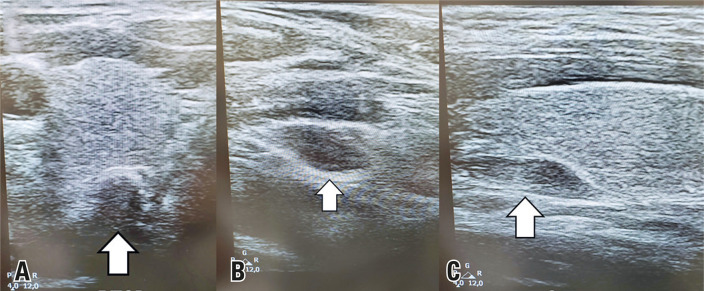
Preoperative ultrasound showing three parathyroid glands. (A) Arrow indicating the right superior parathyroid gland; (B) arrow indicating the right inferior parathyroid gland; (C) arrow indicating the left superior parathyroid gland
